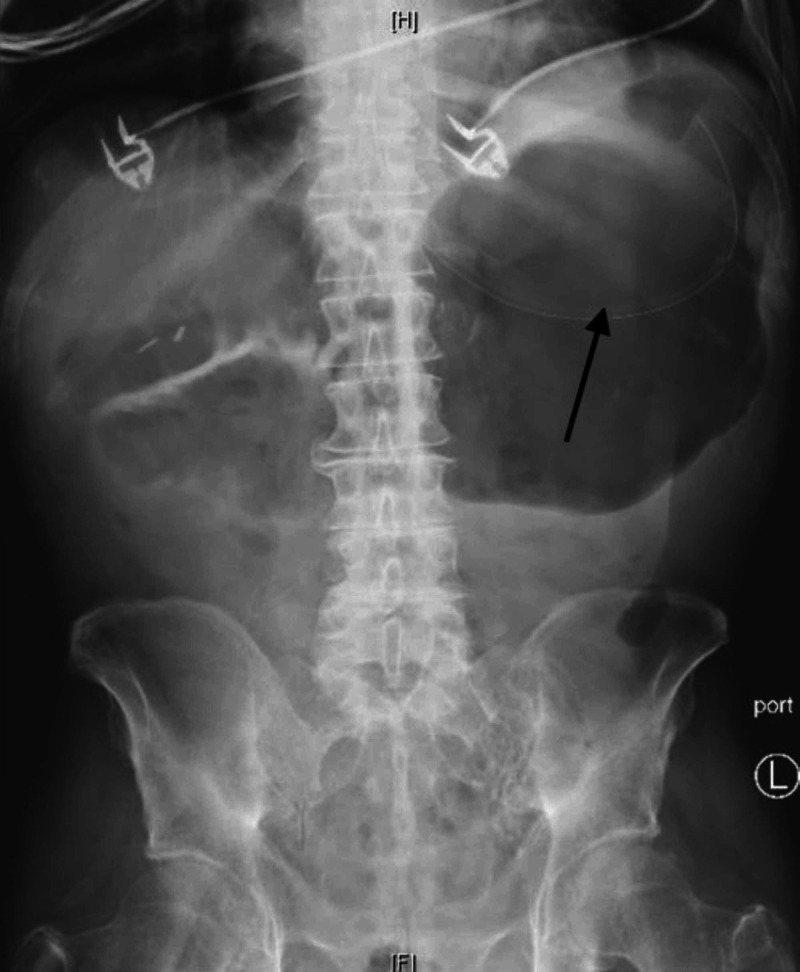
Marked gaseous distension of the stomach seen on abdominal X-ray
